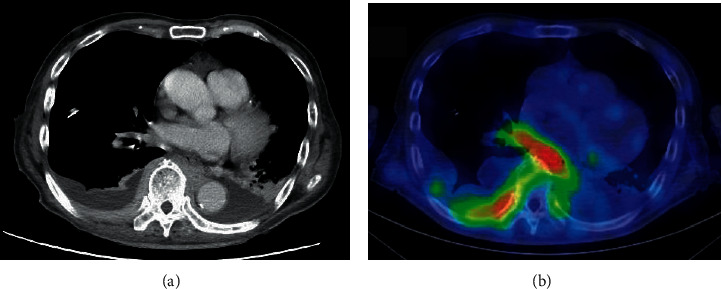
Image of pleural lymphoma. (a) Enhanced computed tomography. (b) Gallium scintigraphy.
